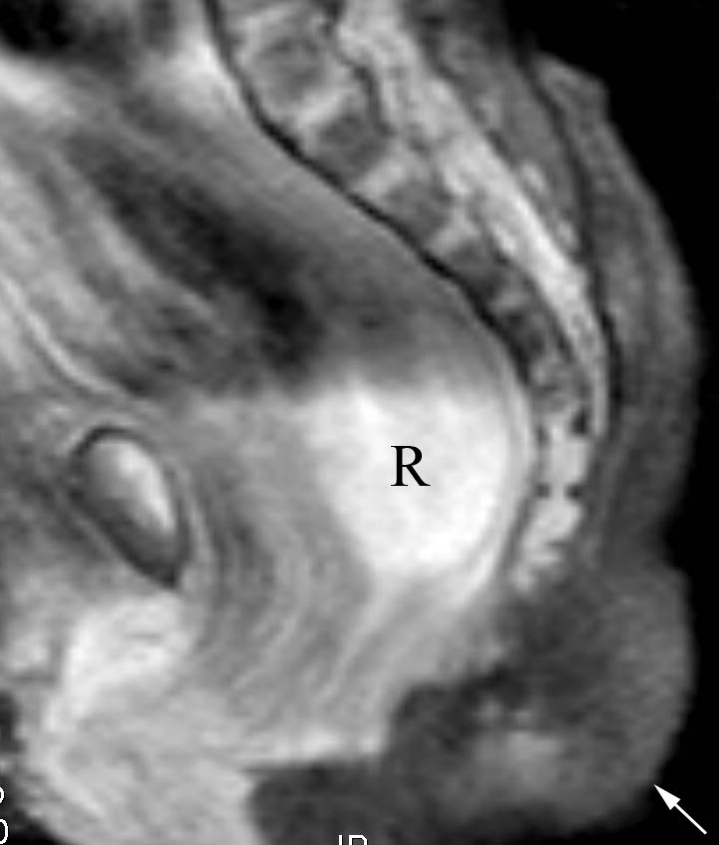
Magnetic resonance imaging of pseudotail and sacrum. This T2 fat-saturated coronal image demonstrates absence of a presacral mass, relatively normal appearance of the sacrum, and dilated rectum (R) in this child with known imperforate anus. The fat containing pseudotail is incompletely included (arrow).
